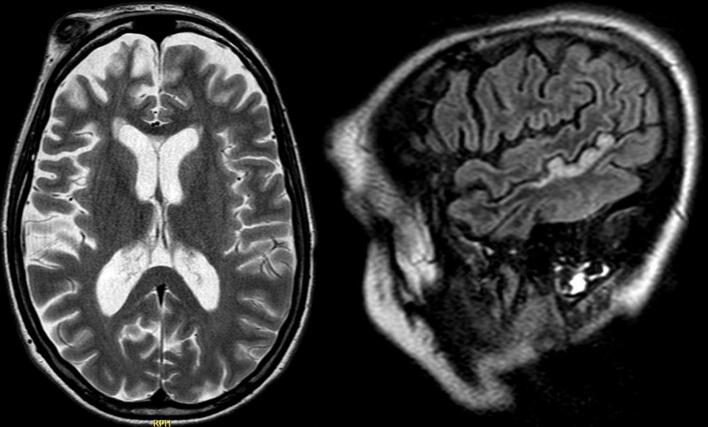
Brain MRI showed global frontal atrophy and T2 hyperintensities in the right MCA territory, consistent with postictal changes, with no new structural abnormalities.
